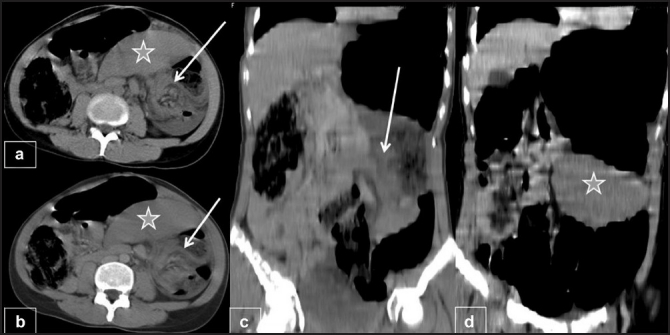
Contrast enhanced abdominal CT in axial (a, b) and sagittal views (c, d) showed spleen vessels and surrounding fat forming a whirled appearance (arrows) at the spleen (star) hilum. The spleen is located below the level of the left kidney
